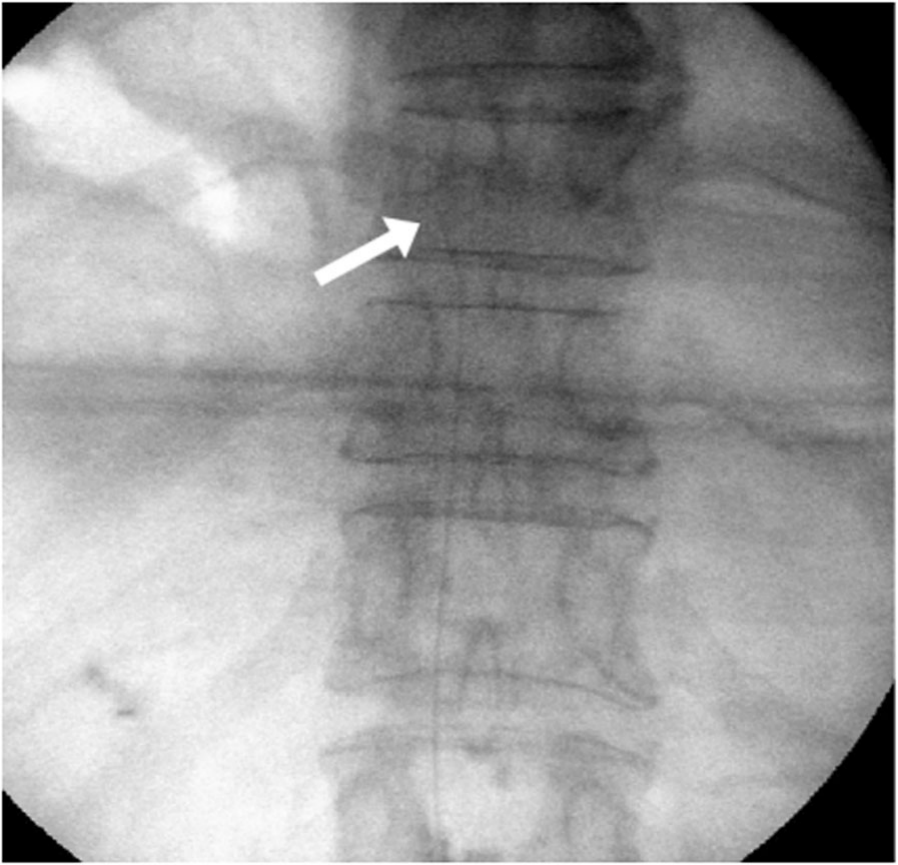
Fluoroscopic images of continuous epidural block using the EpiStim catheter. This catheter has a built-in conductive guidewire that allows the detection of the location of the catheter tip using radiography along with electric stimulation. The arrow indicates the guidewire in the EpiStim catheter
